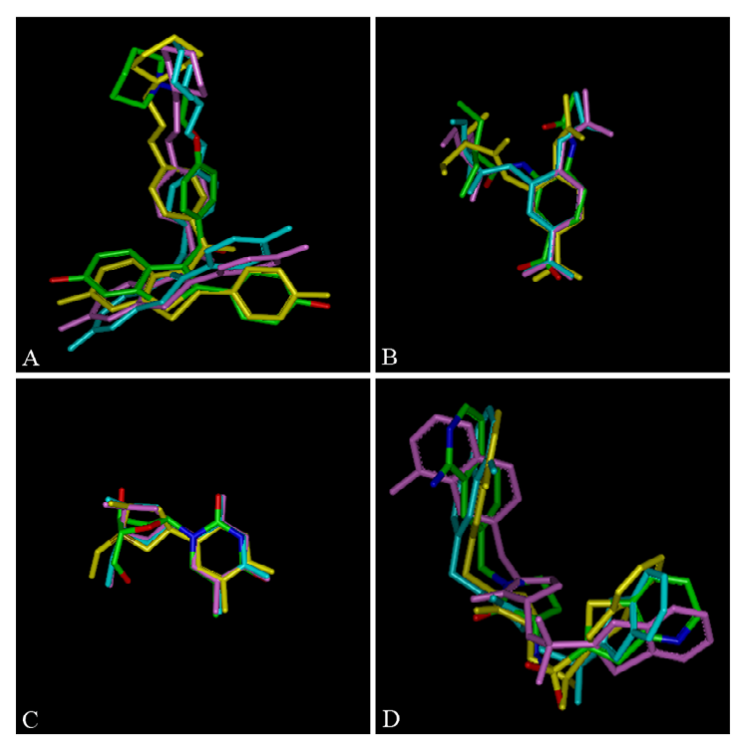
Structural refinement of four small molecules with AMMOS (magenta), MMFF94s (cyan) and Tff (yellow). The minimized structures are superimposed on the corresponding X-ray structures (all atom colored). Raloxifene (A), FDI (B), thymidine (C) and PR2 (D).
